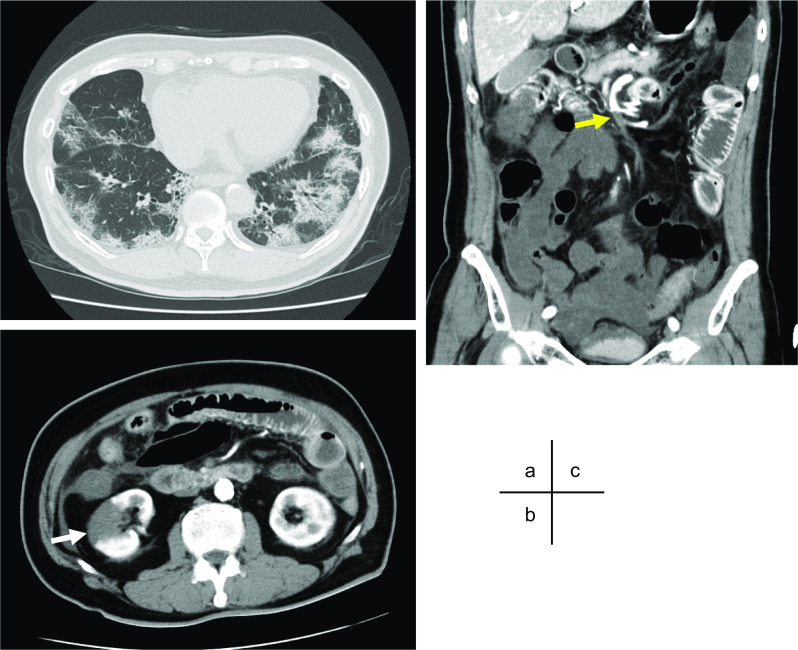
Contrast computed tomography (CT). Contrast CT revealed ground glass opacification with distribution suggestive of COVID-19 pneumonia (a), right renal infarction (b, white arrow), and superior mesenteric artery occlusion (yellow arrow) with intestinal ischemia suggesting extensive necrosis from the jejunum to the transverse colon (c)
